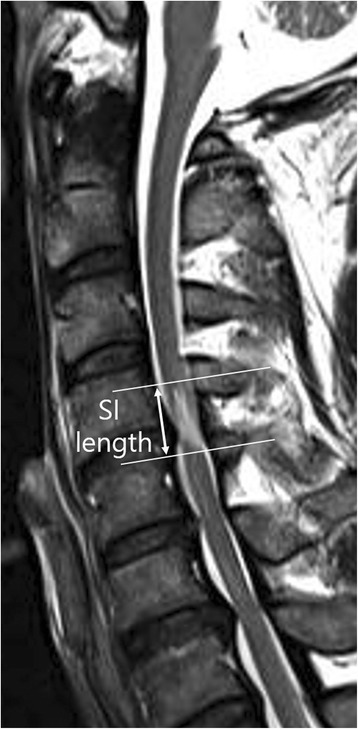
Length of signal intensity. It was measured by the proximal-distal range of the intramedullary hyperintensity on T2-weighted images
